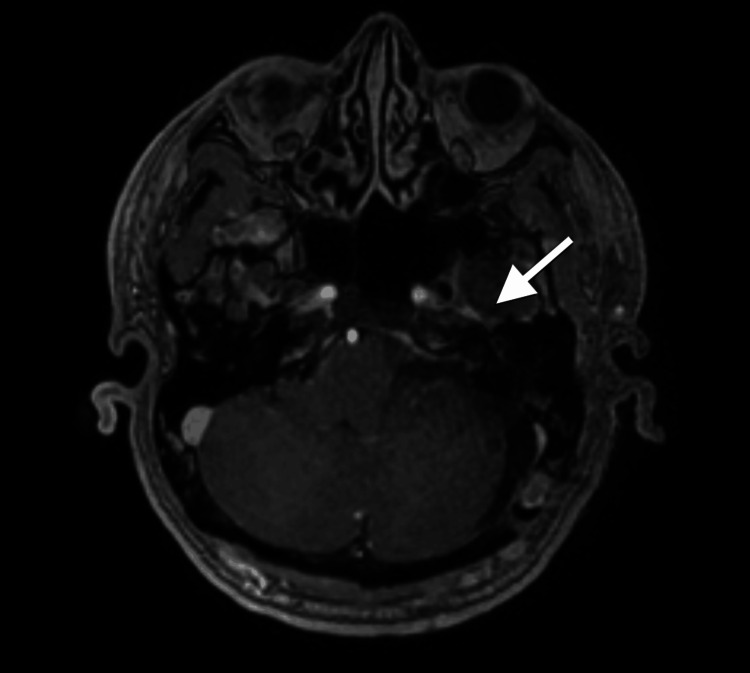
Postoperative MRI (T1-weighted with contrast) demonstrating complete tumor resection (white arrow)
